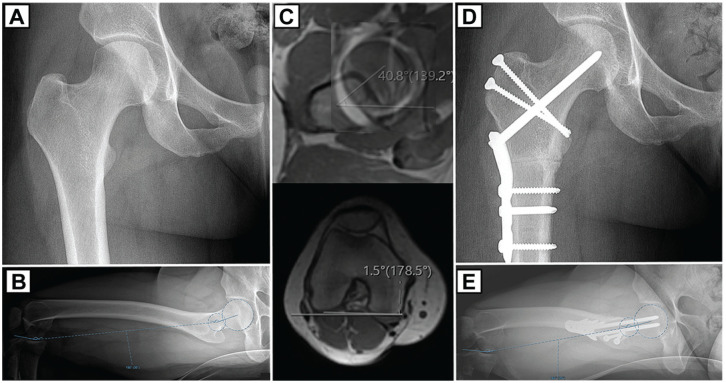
(A) Anteroposterior pelvis radiograph obtained in a 25-year-old female patient with right hip pain. (B) The modified Budin view demonstrates a femoral anteversion of 30°, suggestive of increased femoral version. (C) Magnetic resonance imaging confirmed an increased femoral version of 43° according to Murphy. (D) Postoperative anteroposterior pelvis radiograph obtained after subtrochanteric derotation osteotomy via surgical hip dislocation. (E) The postoperative modified Budin view enables quantification of the correction, with postoperative femoral version measuring 20°.
